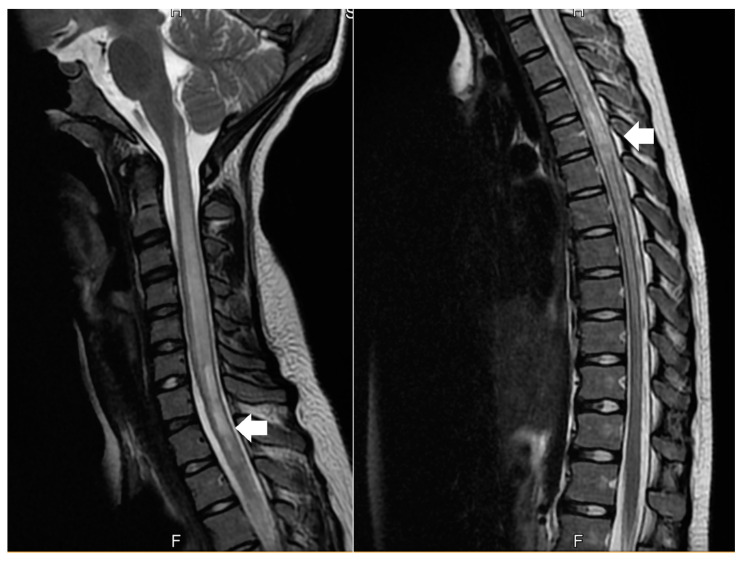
Spinal MRI showing cervical and thoracic (C6-T8) hyperintense lesions on T2-weighted imaging (White arrows).
